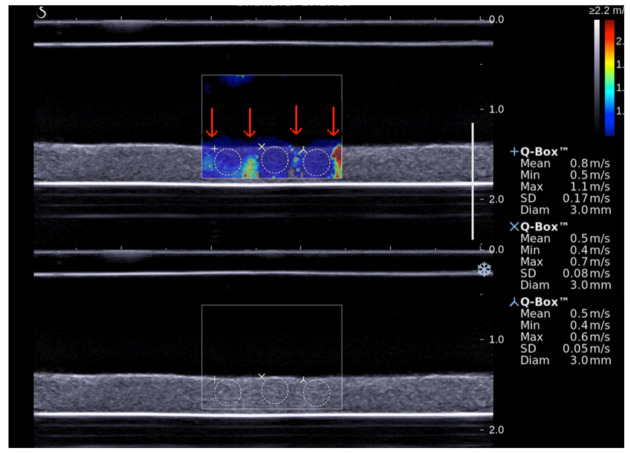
Shear wave ultrasound imaging of blood clot. Red arrows show ultrasound beam push artifacts. SWS was measured with Q-box^®^ software.
